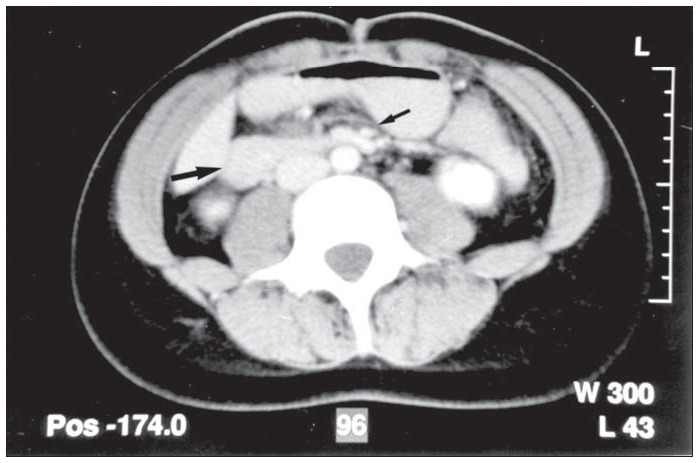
Abdominal computed tomography after prolonged hypercaloric parenteral nutrition showing absence of duodenal distension (large arrow) and replacement of the retroperitoneal adipose stocks at the level of the aortomesenteric constriction (small arrow).
